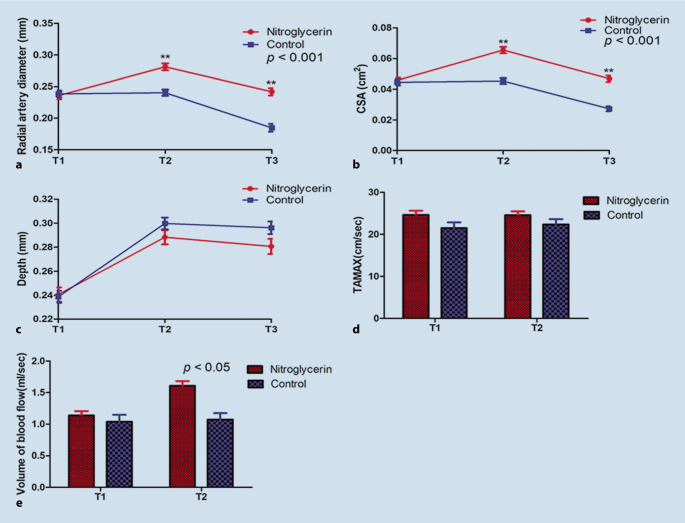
Results of ultrasonographic measurements: a radial artery diameter (mm), b cross-sectional area (CSA, cm^2^), c depth (mm), d time average maximal velocity (TAMAX, cm/sec), e volume of blood flow (ml/sec). T1 = baseline; T2 = 3 min after subcutaneous injection; T3 = immediately after radial artery cannulation
